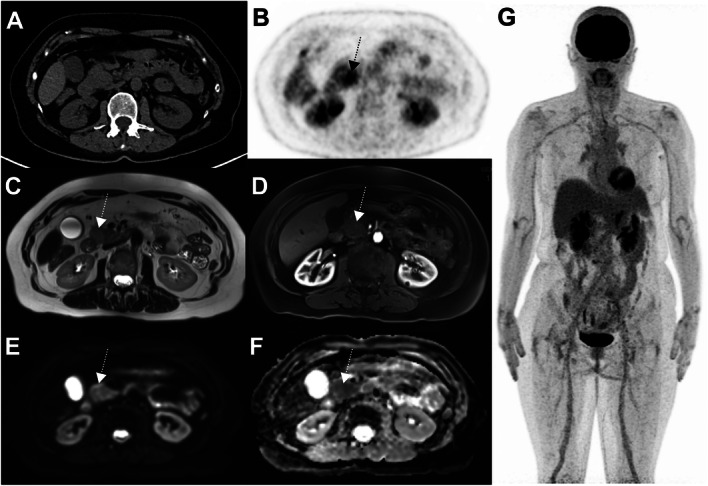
63-year-old woman with prior localization of melanoma on the left thigh, who had been treated by ICIs for 16 months. A nodular 18F-FDG focus can be seen in the pancreatic head (B: axial PET slice) without concordant anomaly at CT (A). Given that this pattern was unusual, MRI was performed a week later and showed patterns consistent with pancreatic adenocarcinoma: ill-defined mass of the pancreatic head on T2 sequence (C), this lesion appearing hypointense on T1 sequence arterial phase after gadolinium injection (D), hyperintense on diffusion sequence and displaying hypercellularity on ADC sequence. Given that PET was otherwise normal, a biopsy was performed and confirmed pancreatitis. Subsequent PET showed spontaneous recovery (data not shown)
